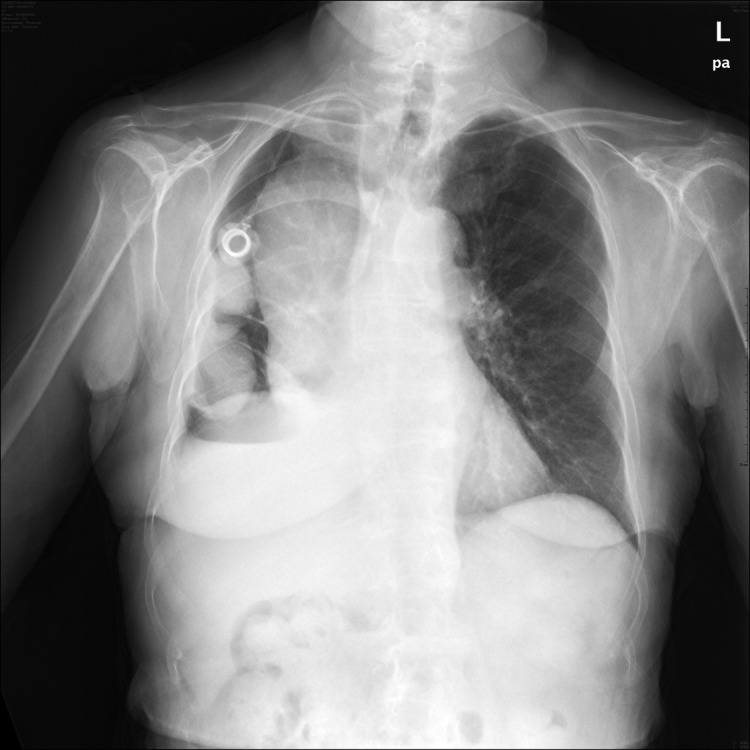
Chest X-ray of a 77-year-old female with a malignant solitary fibrous tumor showing three large masses in the right pleural muscles.
